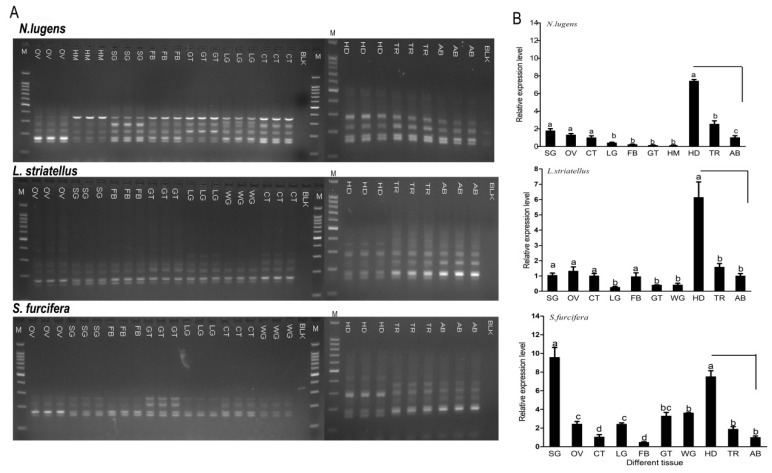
(A) RT-PCR products of cDNA templates prepared from total RNA prepared from different tissues and parts of *N. lugens*, *L. striatellus,* and *S. furcifera*. RT-PCR amplified with primer CaMKII-VR based on the common sequences around the variable region in *CaMKII*. (B) RT-qPCR analysis of cDNA templates prepared from total RNA prepared from different tissues and parts of *N. lugens*, *L. striatellus*, and *S. furcifera*. The RT-qPCR was analyzed with specific primer qCaMKII, which corresponded to identical nucleotides in the catalytic domain of *CaMKII*. The mRNA levels for each cDNA were normalized with respect to the 18s-rRNA mRNA level. Fold induction values of *CaMKII* genes were calculated with the ΔΔ*C*t equation and normalized to the mRNA level of *CaMKII* genes in the tissue cuticle or abdomen which were defined as 1.0. Each point represents the mean ± SE from three independent experiments. HM: hemolymph; FB: fatbody; SG: salivary glands; GT: midgut; OV: ovary; CT: cuticle; LG: leg; WG: wing; HD: head; TR: thorax; AB: abdomen and BLK: blank control with H_2_O as the template. As we could not obtain enough of a sample for the hemolymph extraction from either *L. striatellus* or *S. furcifera* and as the wings had been dissected from *N. lugens*, there are no expression data for these tissues.
